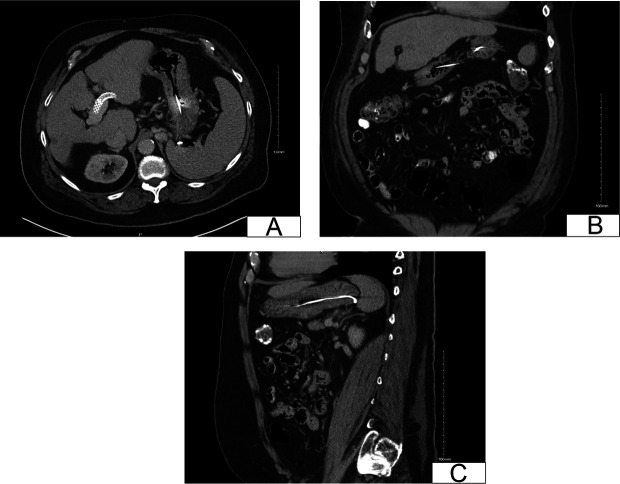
Computed tomography scan (CT) of the abdomen/pelvis showing a thin-metallic object within the duodenum and stomach. Transverse view (A), coronal view (B), and sagittal view (C).
